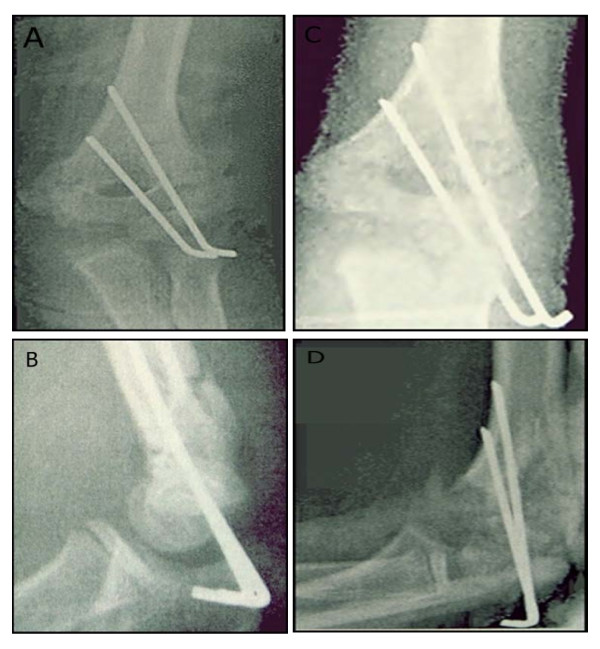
A, Immediate postoperative Anteroposterior radiograph of two-lateral entry pinning showing acceptable reduction. B, Immediate postoperative lateral radiograph of two-lateral entry pinning showing acceptable reduction. C, Follow-up Anteroposterior radiograph showing loss of reduction in two-lateral entry pinning. D, Follow-up lateral radiographs showing loss of reduction in two-lateral entry pinning.
